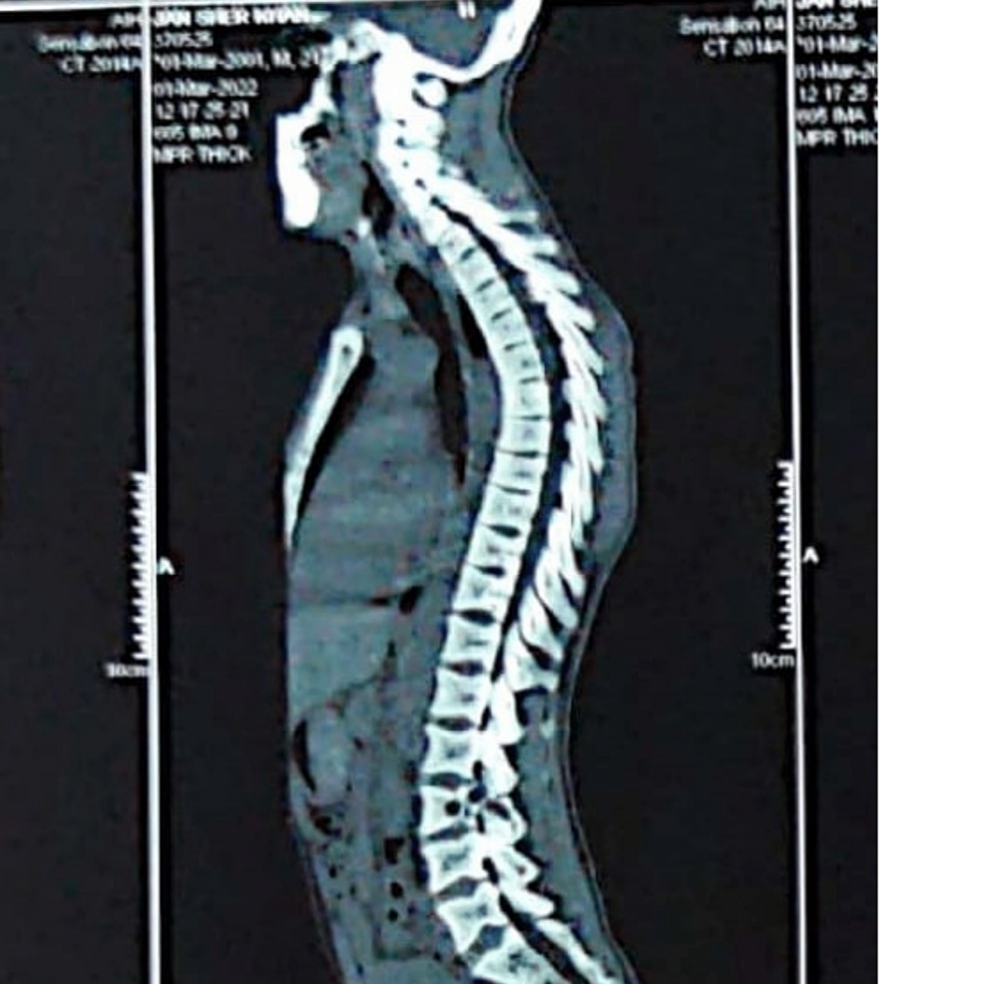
MRI spine without contrast suggestive of a complete collapse of the C3 vertebral body and partial collapse of the C4 vertebral body. There is accentuation of the lordotic curvature.
